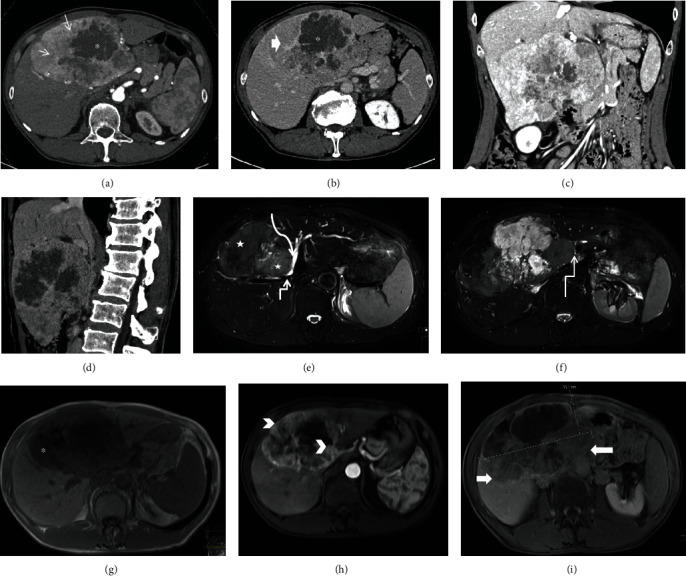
CT scan (a–d) showed a large multiloculated, partly nonvascularized (^∗^), partly hypervascularized (arrows) tumor with washout (thick arrow). MRI (e–i) confirmed a 15.5 × 9.6 × 14.2 cm tumor which was T1 hypointense (^∗^) and heterogeneously T2 hyperintense (stars) and displayed varying levels of peripheral enhancement on postcontrast sequences (arrow heads) with washout (thick arrows). The mass compressed the central and extrahepatic bile ducts (angulated arrows) with dilated intrahepatic bile ducts (curved arrow).
